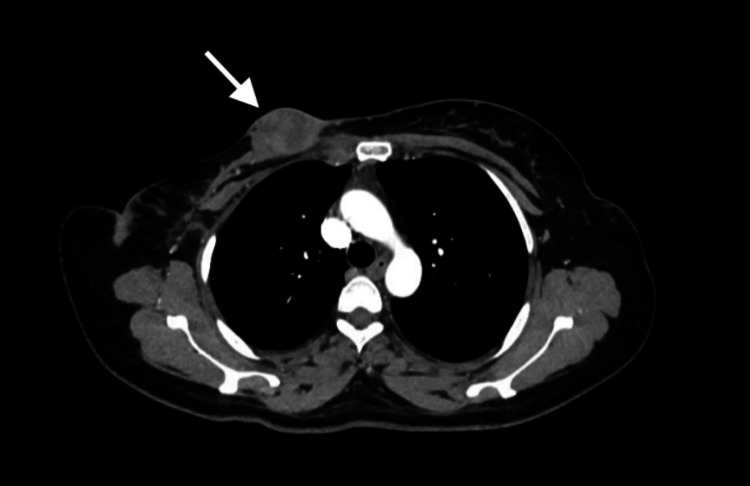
Horizontal section of computed tomography showing growing lesion on the surgical site invading the skin (arrow), indicating locoregional recurrence post right modified radical mastectomy
